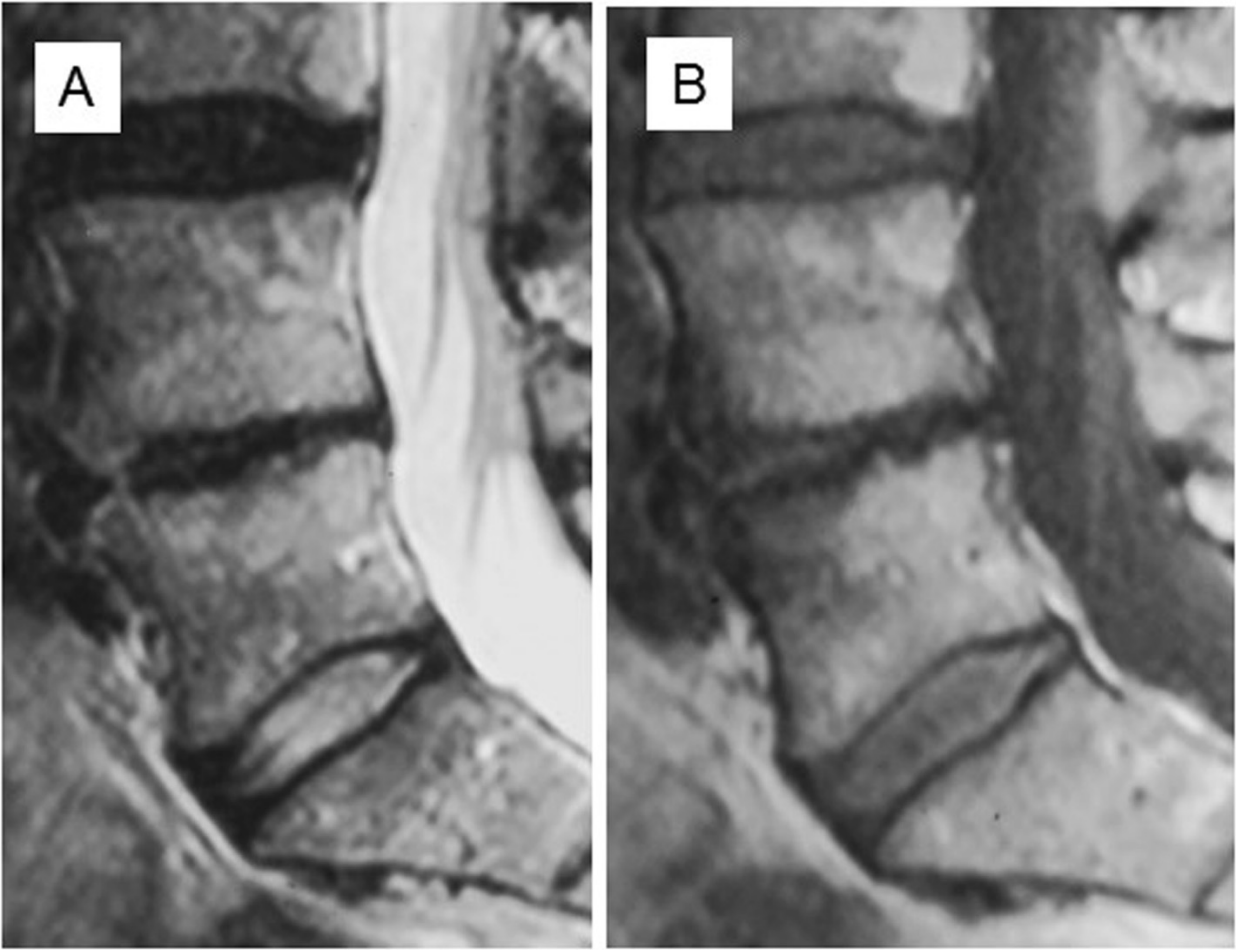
T2 (A) and T1 (B) -weighted sagittal MR images at follow-up of the same patient as in Figure 1 showing that the Modic change has converted to Type 2. Low back pain intensity 2/10, Oswestry Disability Index 12%.
